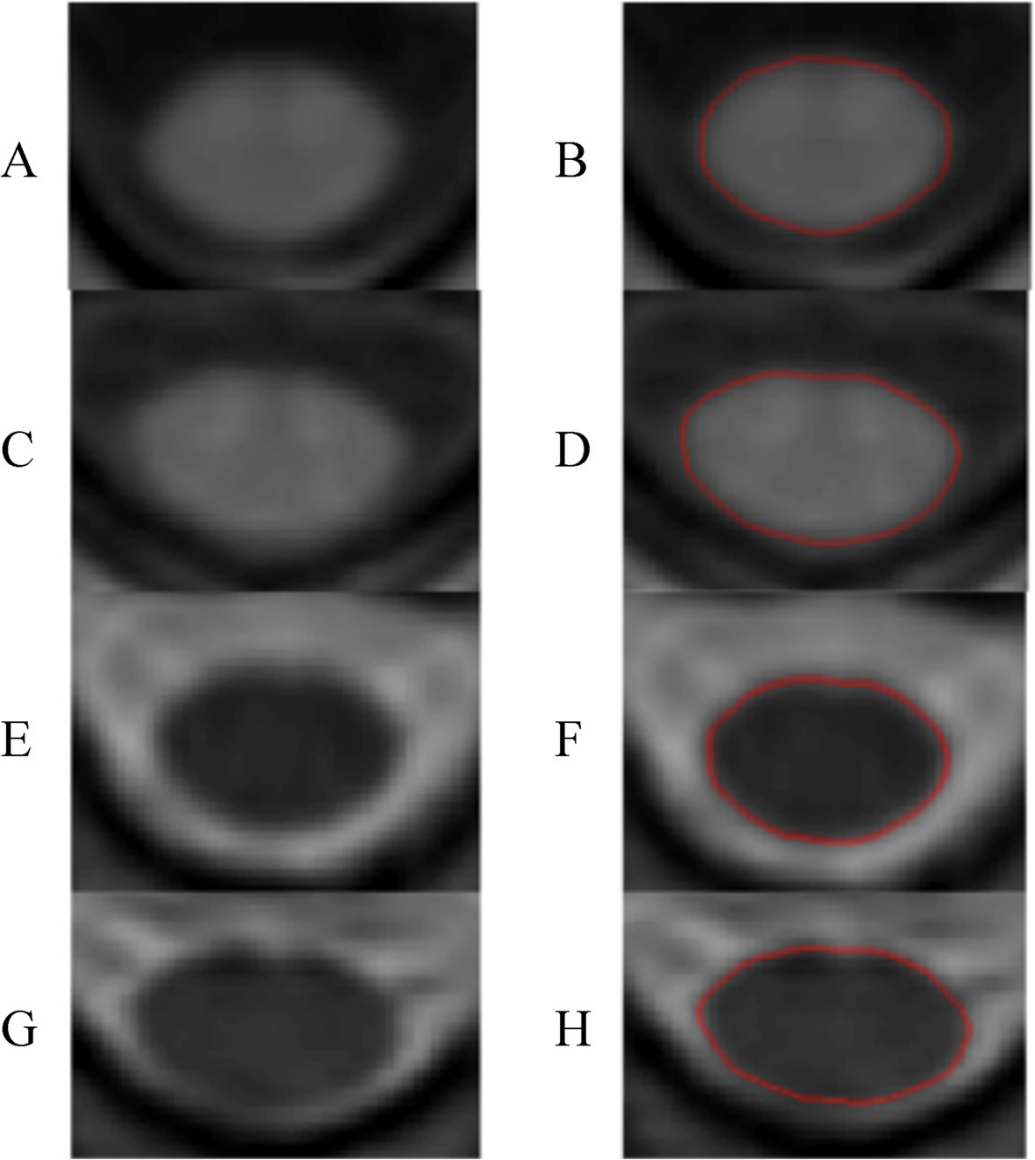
T1-weighted gradient echo (a–d) and T2-weighted fast spin-echo (e–h) axial MRI scans from C2. Left = raw images; right = cord outlines (*red*) after segmentation. a, b, e, f: Patient with relapsing-remitting MS [41 year-old woman, 5.4 years disease duration, low physical disability (EDSS score = 1)]. c, d, g, h: Normal control (49 year-old woman)
